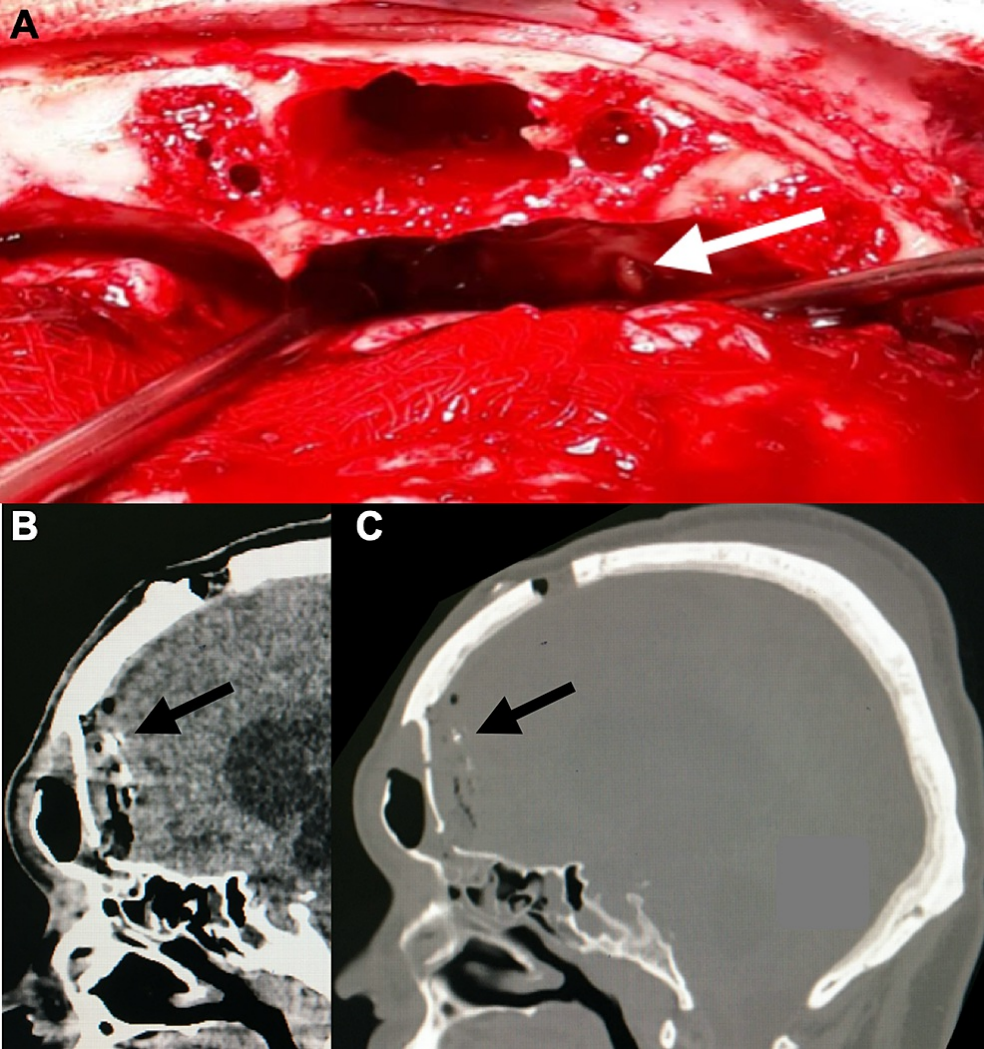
Surgery. (A) Small left frontobasal meningocele (white arrow); (B) CT scan of skull sagittal  showing interposition of the galea aponeurotica in the frontobasal region (black arrow); and (C) bone window showing interposition of the galea aponeurotica in the frontobasal region (black arrow). CT: computed tomography.
